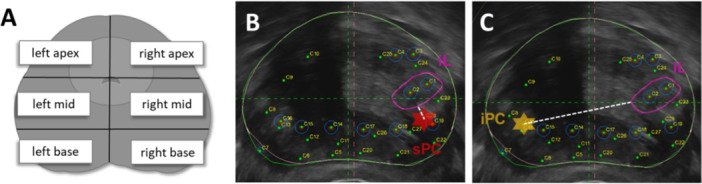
(A) division of prostate in sextants; (B) distance of cancer‐free MRI index lesion to the closest sPC core; (C) distance of cancer‐free MRI index lesion to the closest iPC core. iL, index lesion; sPC, iPC, insignificant prostate cancer; significant prostate cancer. [Color figure can be viewed at wileyonlinelibrary.com]
